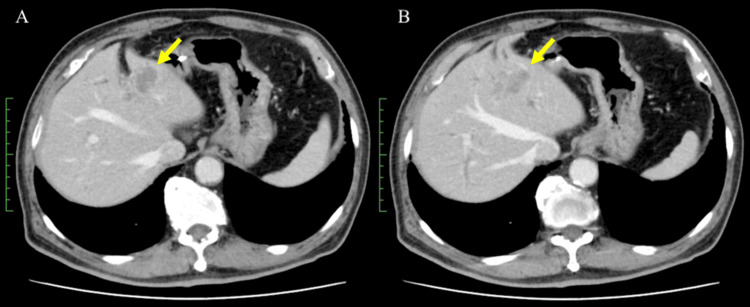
Abdominal computed tomography (CT) images obtained after three cycles of treatment with cisplatin, gemcitabine, and durvalumab (A, B) Abdominal CT images revealed an enlarged primary tumor in the liver (arrow).
